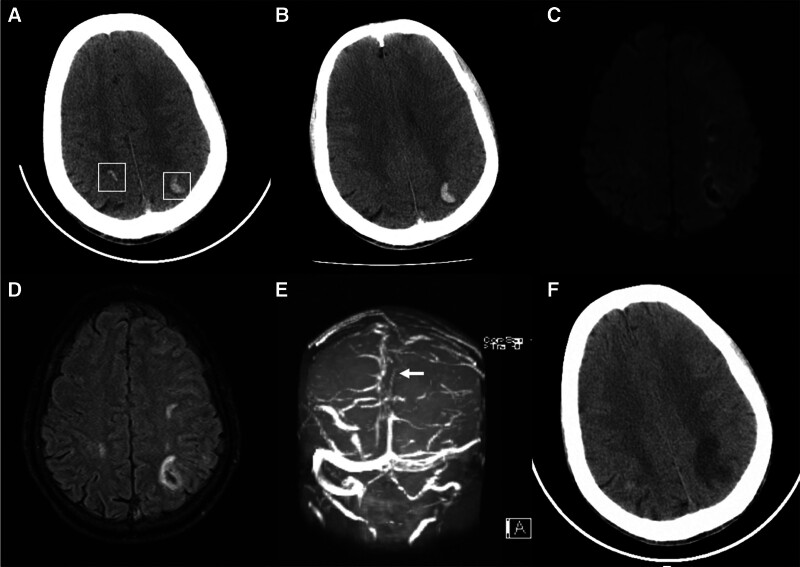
Case 2. (A and B) The brain CT scan shows symmetrical crescent-shaped intracranial hemorrhage in bilateral parietal and occipital lobes (White boxes). (C and D) DWI and FLAIR show corresponding multifocal cerebral infarction with hemorrhagic transformation. (E) MRV suggests superior sagittal sinus thrombosis (arrow). (F) CT examination obtained at 1-month follow-up. CT = computed tomography, DWI = diffusion weighted imaging, FLAIR = fluid-attenuated inversion recovery, MRV = magnetic resonance venography.
